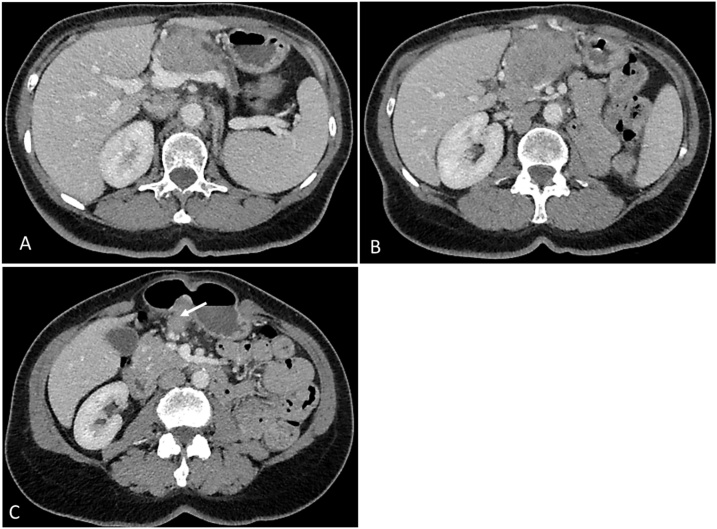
CT-scan images showing the mass of the head of the pancreas and the invasion of the superior mesenteric artery and vein (A & B). (C) Metastatic lymph node (arrow).
